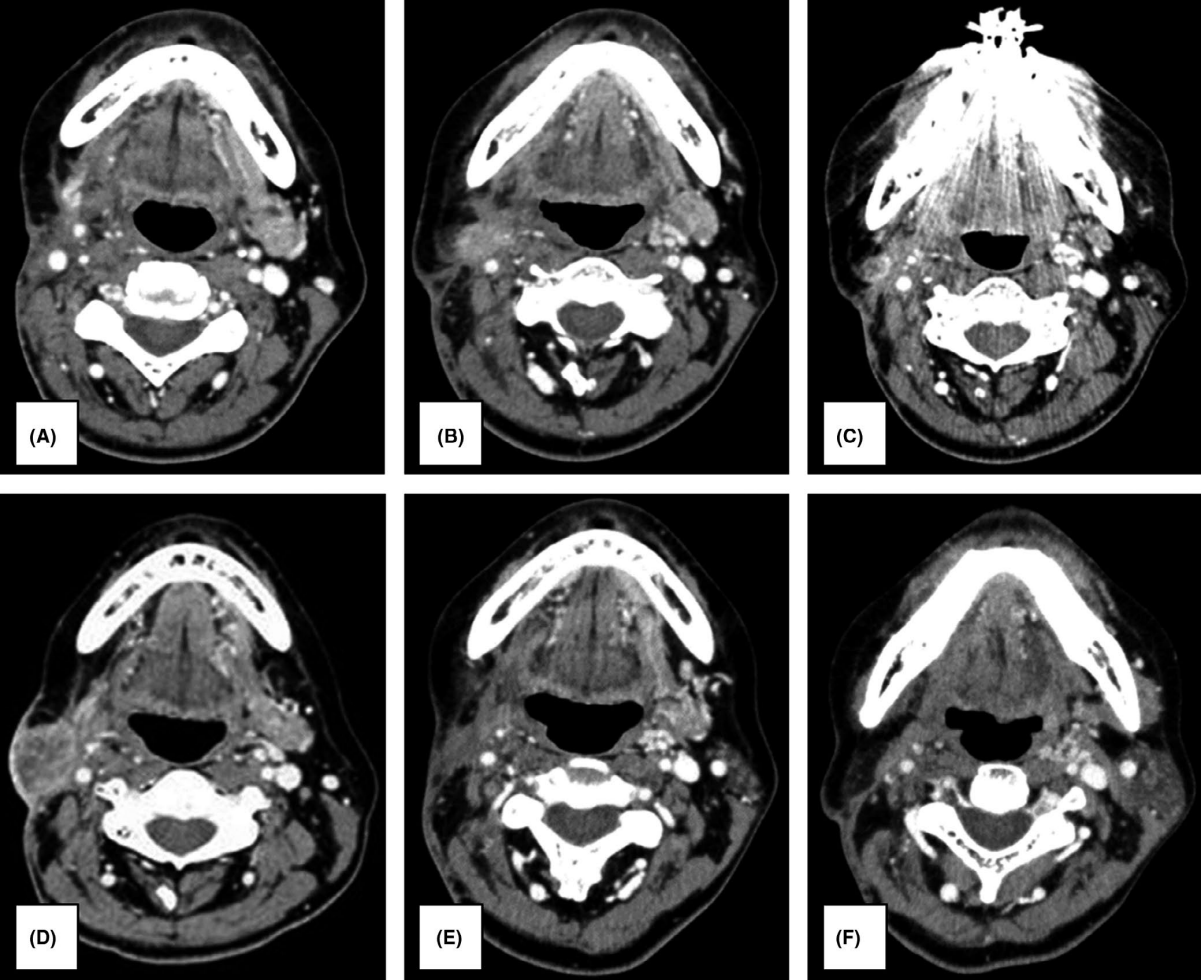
(A) Computed tomography image taken after three courses of cetuximab combination chemotherapy. The right submandibular recurrent lymph node had disappeared. (B) Computed tomography image taken 3 months after the maintenance therapy of cetuximab alone was initiated. Swelling of the right submandibular lymph node was observed. (C) Computed tomography image taken after six doses of nivolumab. Shrinkage of the right submandibular lymph node was observed. (D) Computed tomography image taken after 14 doses of nivolumab. Significant enlargement of the right submandibular lymph node was observed. (E) Computed tomography image taken after three courses of chemotherapy with cetuximab. Significant reduction of the right submandibular recurrent lymph node was observed. (F) Computed tomography image taken after 52 doses of cetuximab alone. The right submandibular lymph node had disappeared
